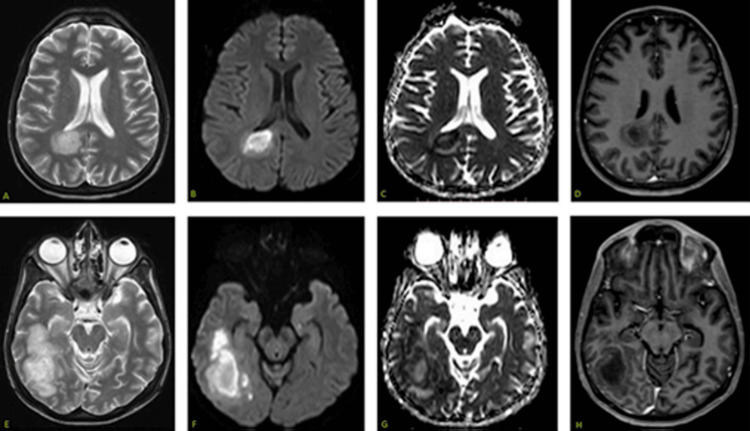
An MRI of the brain with IV contrast shows T2 hyperintense lesions with diffusion restriction and very weak peripheral post-contrast enhancement in the splenium of the corpus callosum extending to right periventricular white matter (A-D), and right temporal lobe (E-H).
